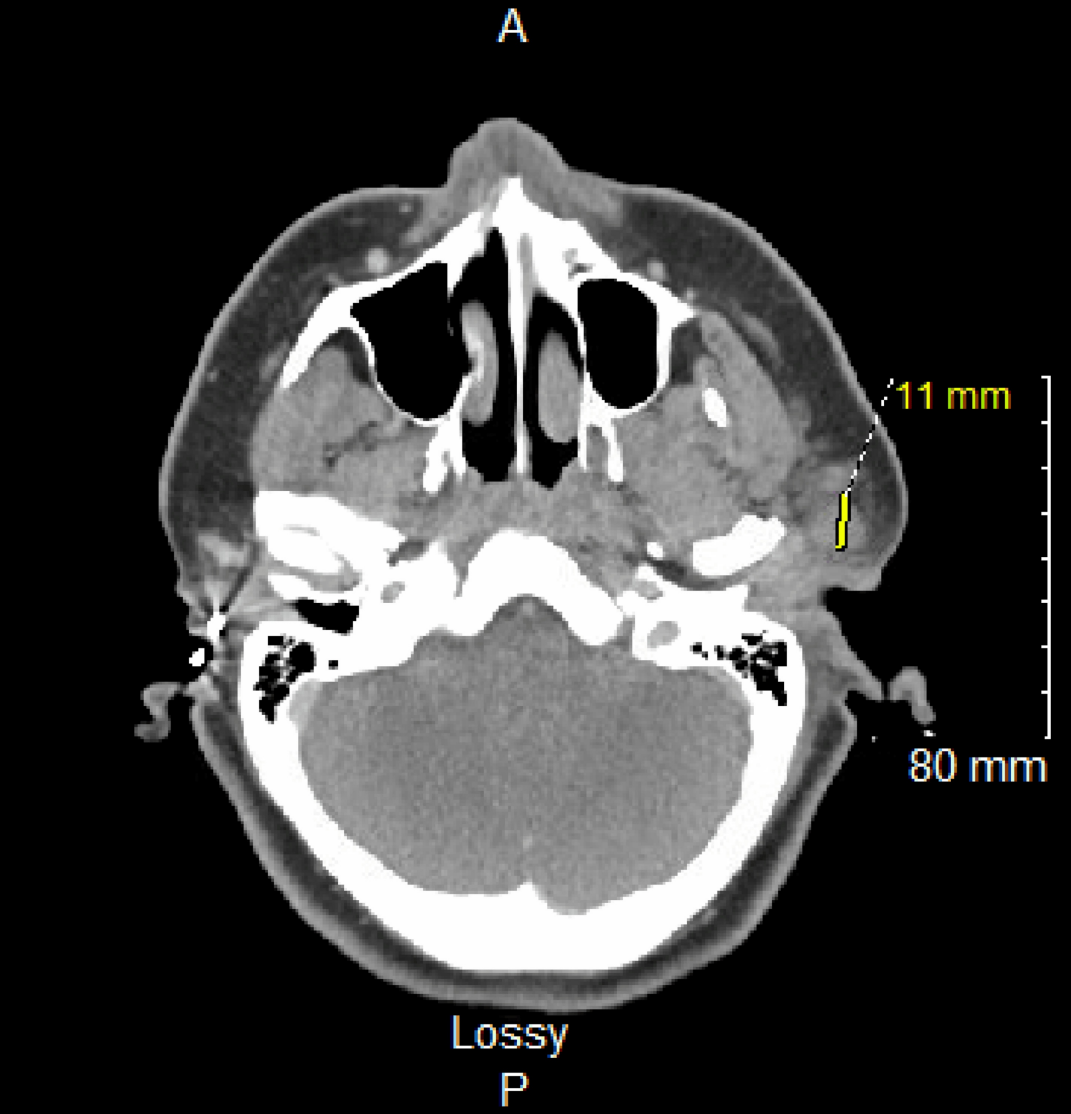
The head and neck CT scan indicated soft tissue density within the left external and middle ear consistent with otitis media and externa with parotid involvement.
